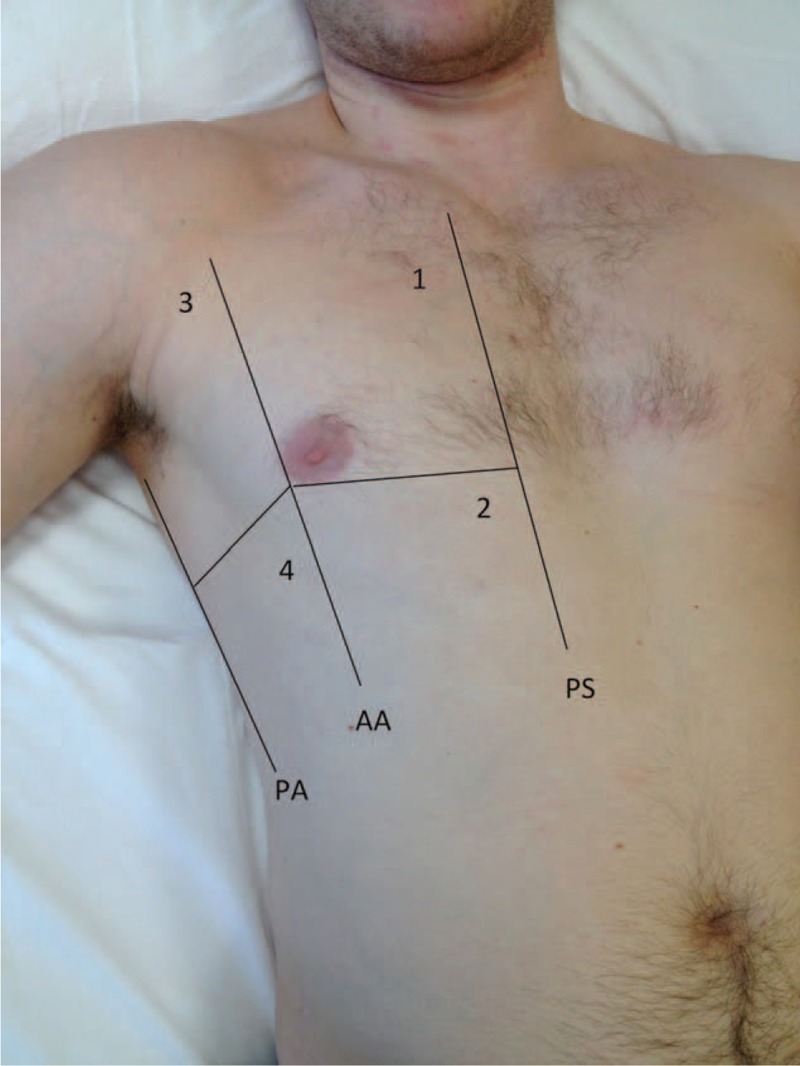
The basic eight-region of the chest for sonographic technique.
